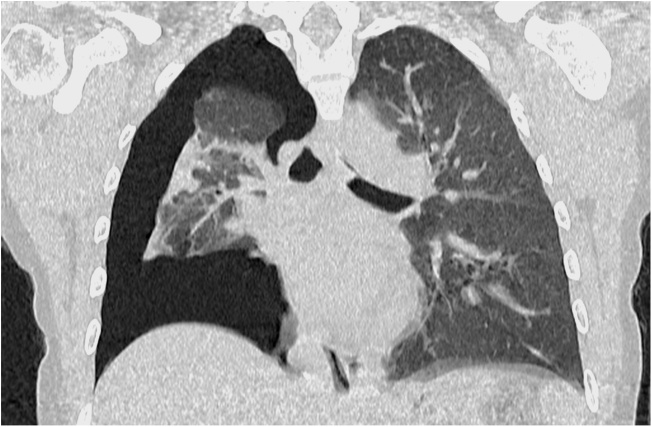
Chest CT showing large right pneumothorax with collapsed lung and pneumomediastinum.
